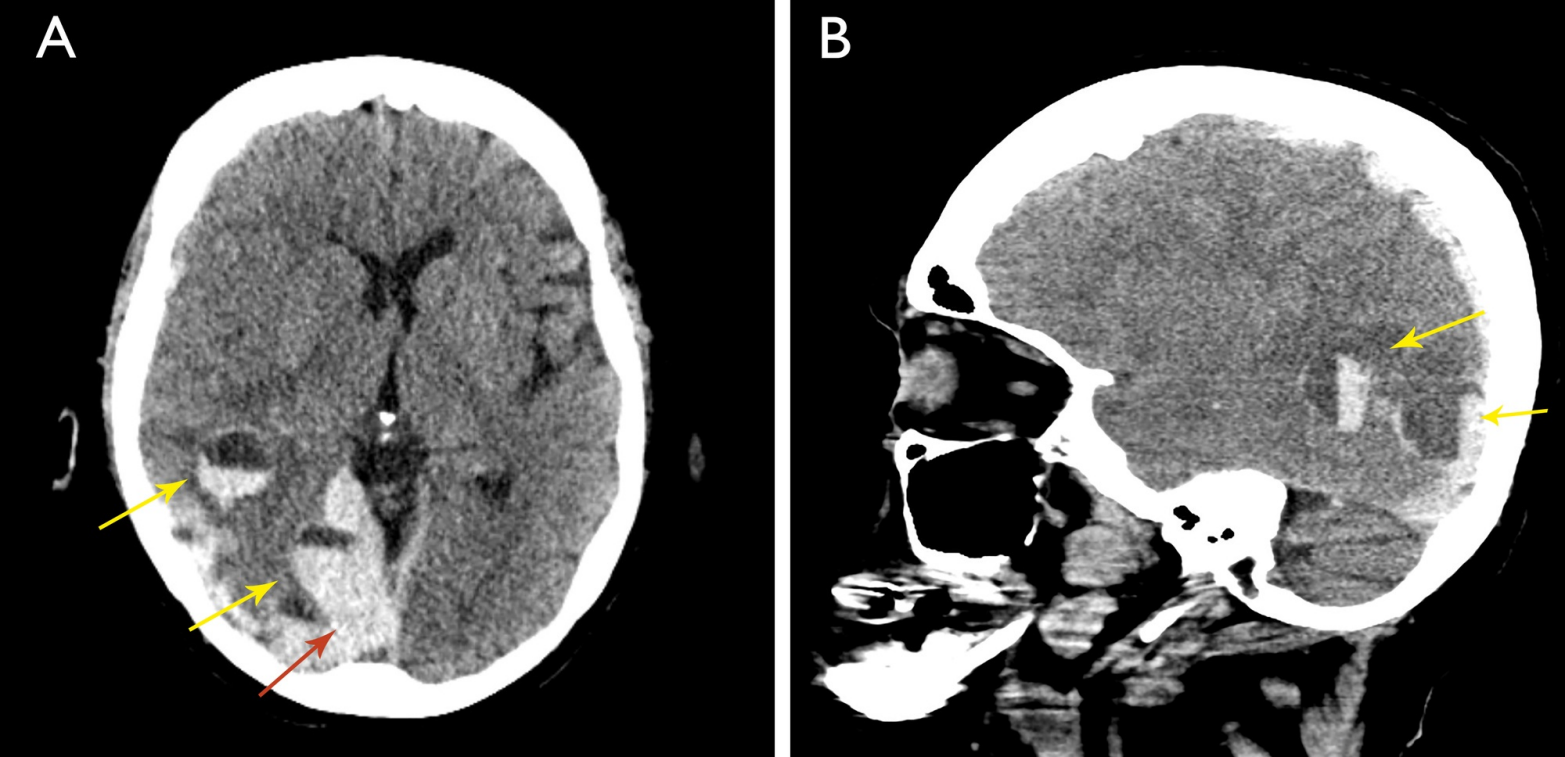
Nonenhanced computed tomography of the brain, with axial view (Panel A) and sagittal view (Panel B), showing an acute supratentorial subdural hematoma (red arrow) and blood fluid levels in right parietal and occipital lobe (yellow arrows).
